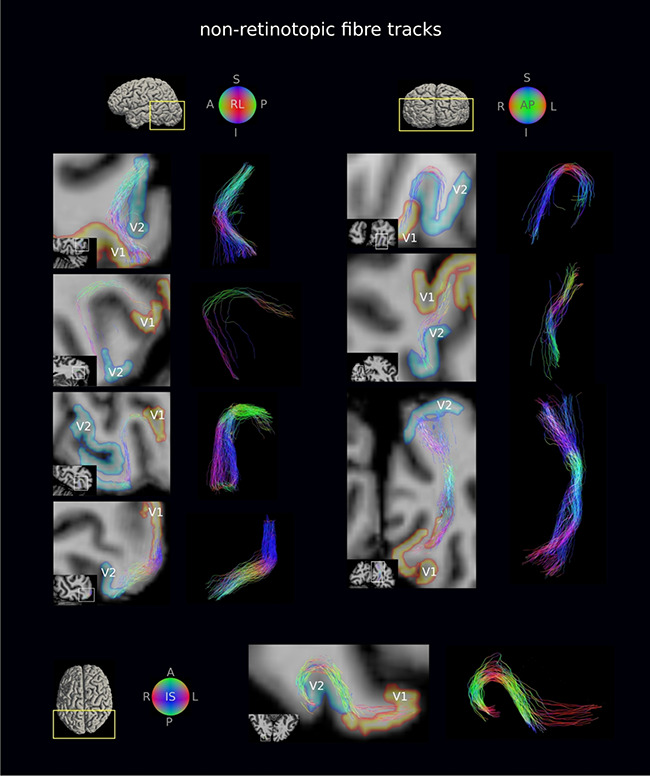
Non-retinotopic V1–V2 fiber tracks were less abundant and on average longer (32 mm mean length) than retinotopic fiber tracks (cf. Fig. 4). A representative subset of non-retinotopic V1–V2 fiber tracks are shown for the flexible surface coil DWI of a representative participant. Fiber tracks are shown on oblique slices and are superimposed on the *T*_1_w image transformed to DWI space. The volumetric V1 and V2 retinotopic segments transformed to the DWI space are also shown. The detected non-retinotopic fiber tracks followed the pattern of cortical folding in many cases, but not all were strictly U-shaped. A, L, R, I, S, P: anterior, left, right, inferior, superior, posterior.
